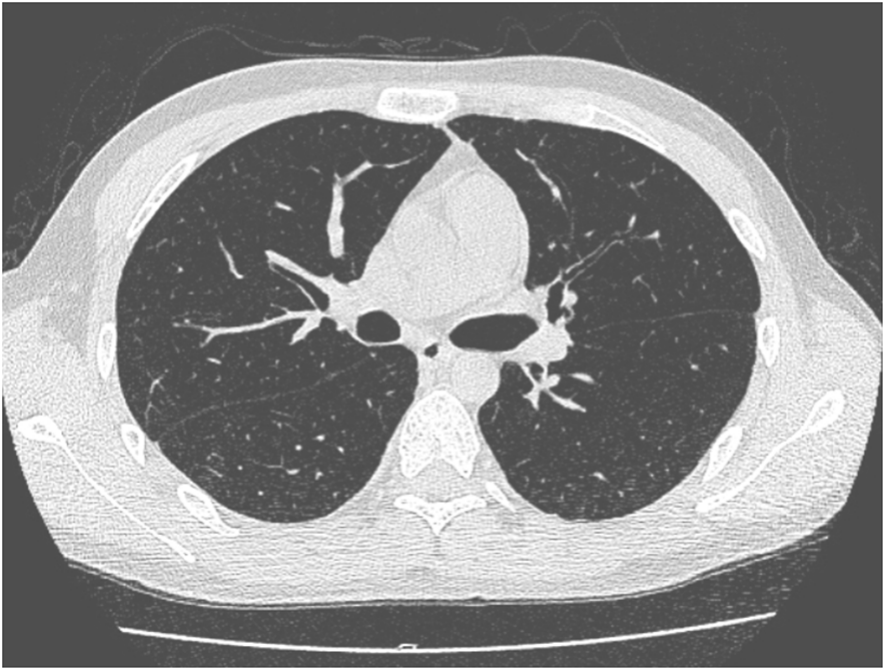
Case 2 lung CT on day −18.
